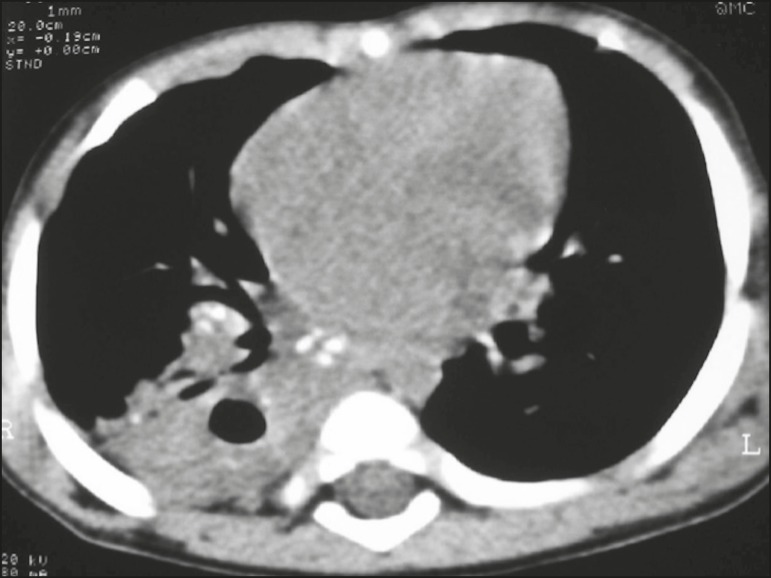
Noncontrast CT, with soft-tissue window settings, of a five-monthold
